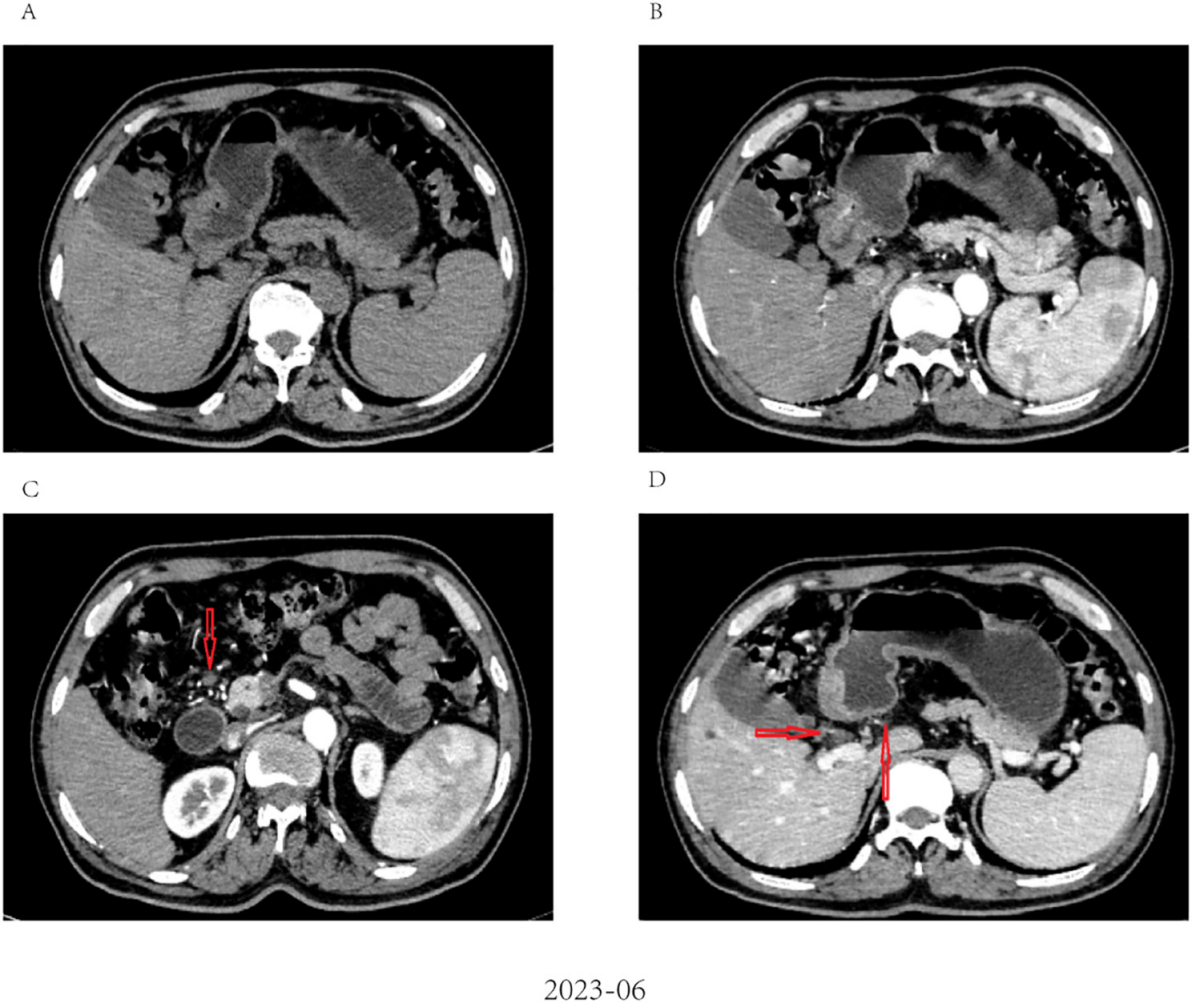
Radiological response evaluation of stomach and abdominal lymph nodes during the clinical course. (A, B) partial response (PR) was confirmed by contrast-enhanced CT, which showed a dramatic reduction in size of tumor after three cycles of tislelizumab combined with SOX; (C, D) Contrast-enhanced CT showed that the size of the abdominal lymph nodes was reduced after three cycles of tislelizumab combined with SOX (red arrow).
